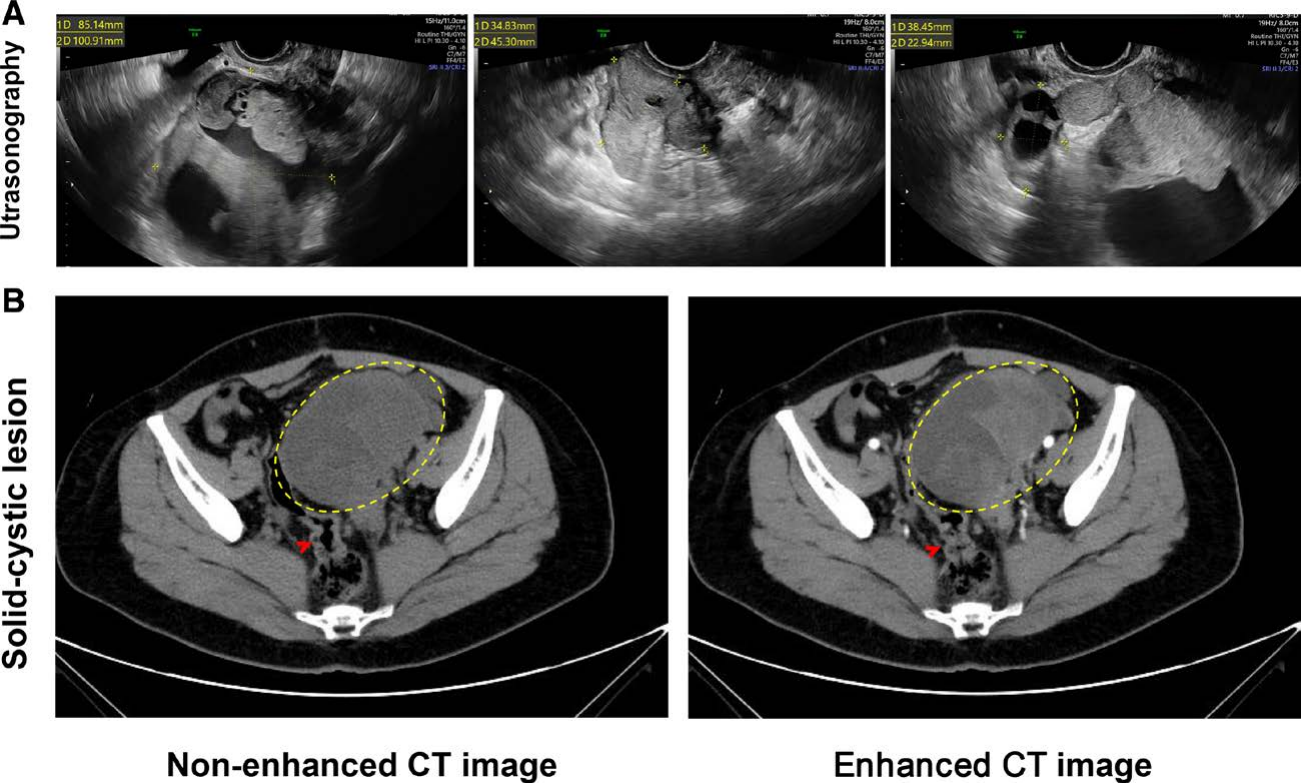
(a) Ultrasonography detected some solid polycystic masses in the pelvic and abdominal cavities (85.1 × 100.9 × 87.4 mm heterogeneous lesion in the pelvis and abdominal cavity, 38.4 × 22.9 × 39.8 mm oval echogenicity in the right pelvis, and 34.8 × 45.3 mm dense echogenicity in the backward of the pelvis). (b) An abdominal and pelvic computed tomography scan demonstrated a solid-cystic lesion of 102 × 96 × 103 mm in the left pelvis, speculated to originate from the left ovary and behaved similarly to ovarian cystic adenocarcinoma. The red arrow points to the thickened sigmoid colon.
